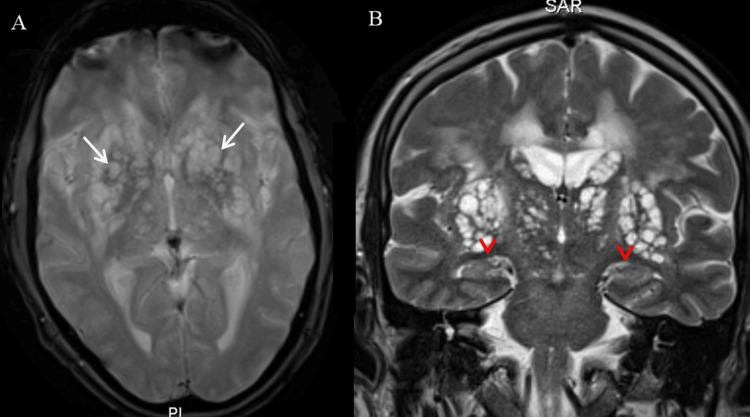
Brain MRI sequences (A) Axial T2*-weighted gradient echo (T2*) image displaying dilated Virchow-Robin spaces (VRS) within the basal ganglia (white arrows), with no hemorrhagic signs or associated magnetic susceptibility artifact. (B) Coronal T2-weighted image at the level of the hippocampal structures (red arrowheads), showing normal hippocampal volume and signal intensity, with no signs of atrophy or hippocampal lesion. The temporal horns of the lateral ventricles remain within normal limits.
